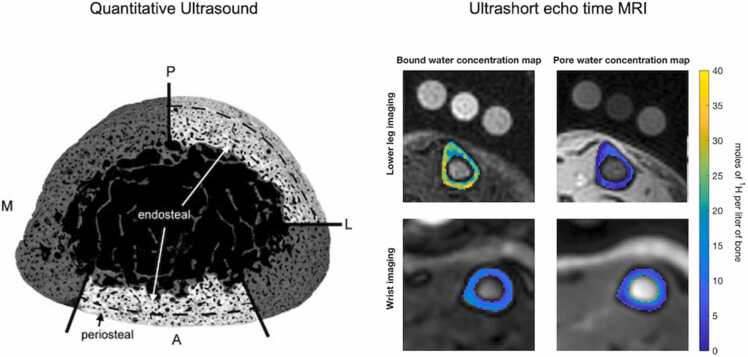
Future directions (A) Acoustic impedance image obtained from quantitative ultrasound (QUS) of an excised radius sample from an adult human. QUS can be used to quantitatively assess bone material properties as it can extract structural parameters of bone with high accuracy. M = medial, P = posterior, L = lateral, A = anterior. (B) Ultrashort echo time (UET) MRI-derived concentration maps for bound water and pore water from 2D scans of the tibia mid-diaphysis (top) and 3D scans of the distal radius (bottom). QUS and UET MRI images adapted with permission from Raum et al., 2005 [93], and Nyman et al., 2023 [98], respectively.
